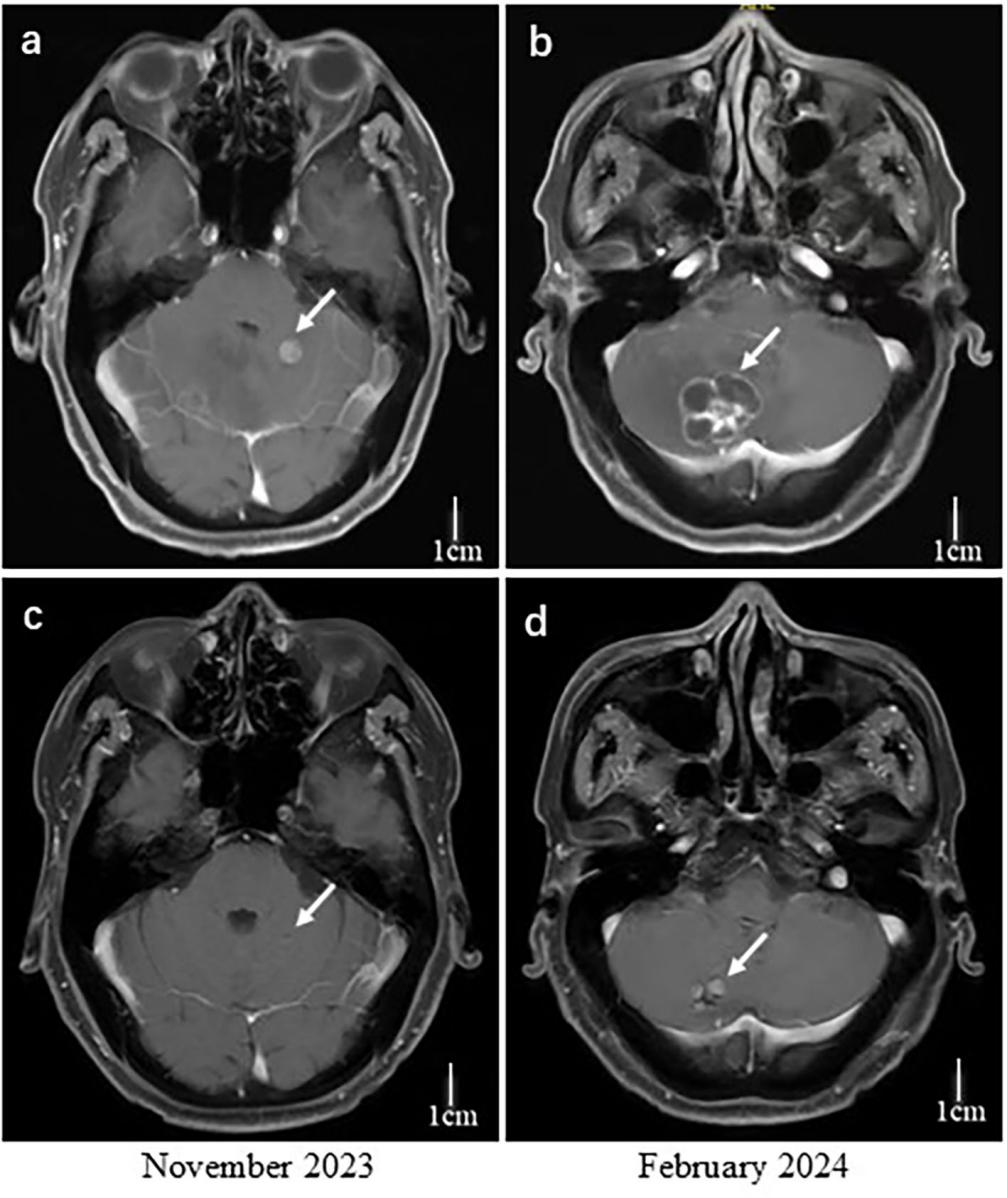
Contrast-enhanced T1-weighted MRI images of the brain before treatment (a, b) and after four cycles of T-Dxd plus bevacizumab (c, d). Scale bars represent one centimeter.
